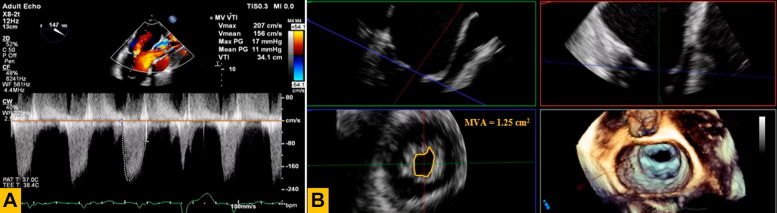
Transesophageal Echocardiography Showing Significant Mitral Stenosis, Patient 1 (A) Mean transmitral gradient of 11 mm Hg. (B) Mitral valve area (MVA) of 1.25 cm^2^ measured by 3-dimensional planimetry.
